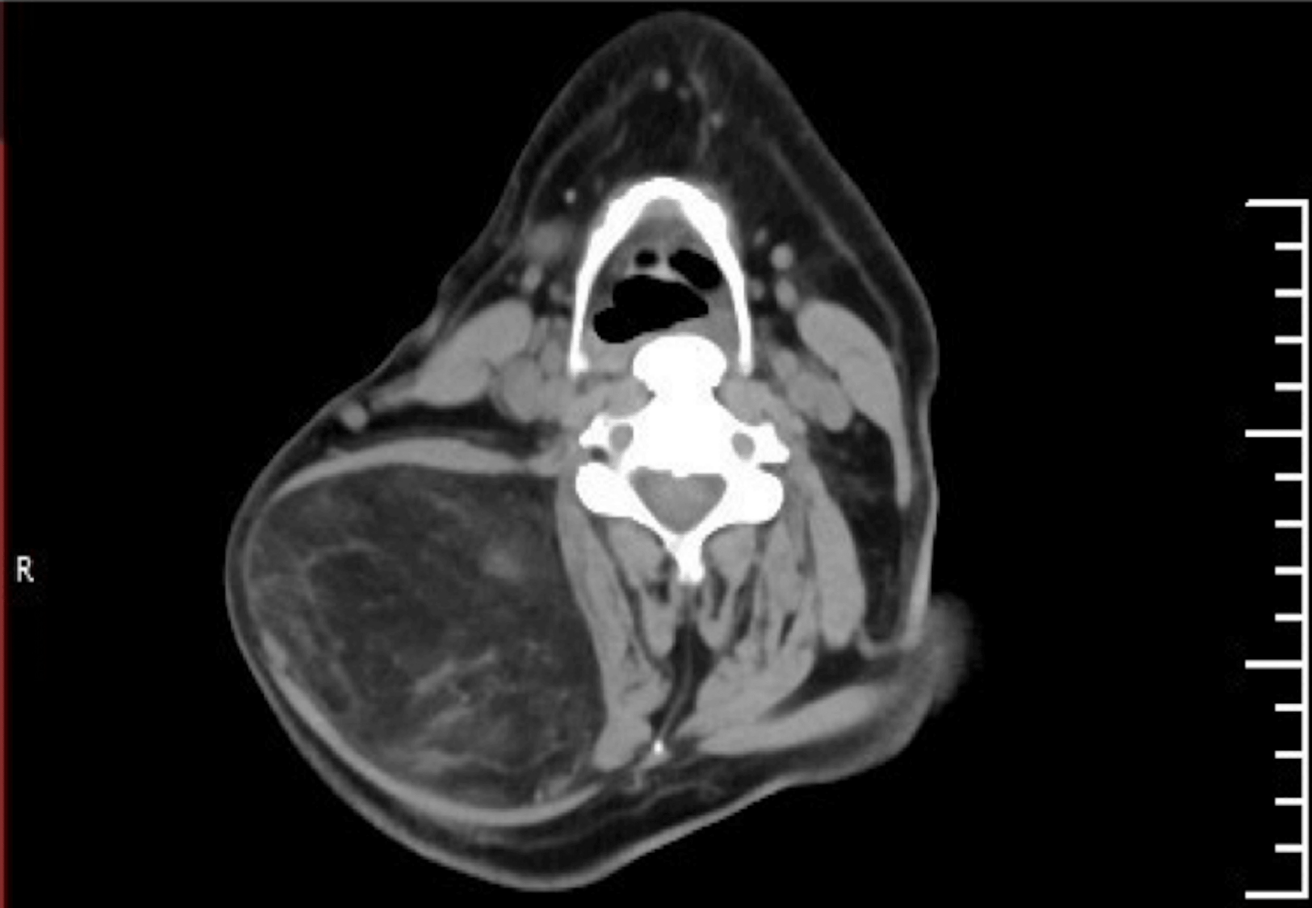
An axial view of the neck CT scan shows a huge right mass.
